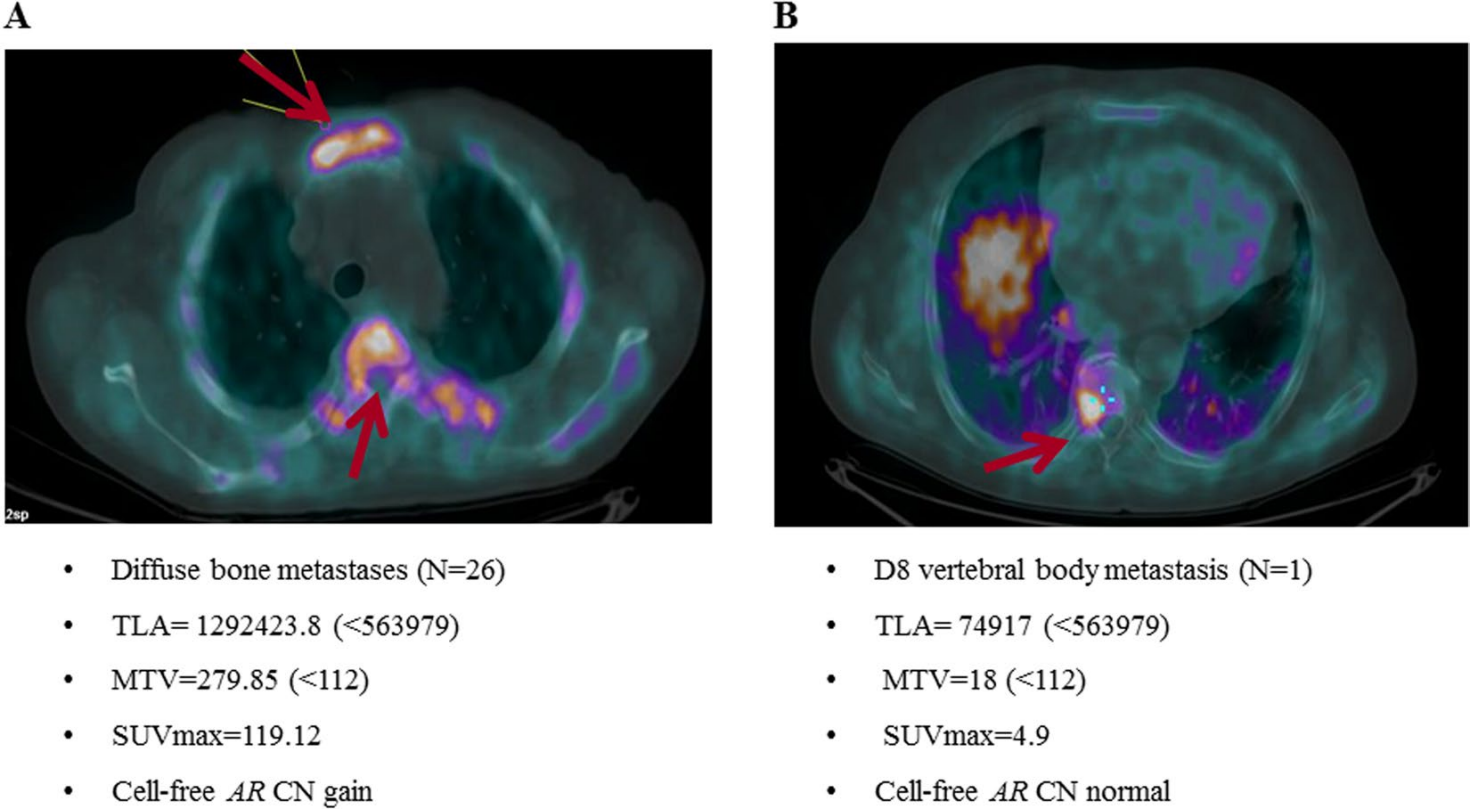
FCH-PET/CT of two metastatic CRPC patients. The FCH PET/CT image (A) demonstrates diffuse metabolically active skeletal metastases involving multiple vertebrae (arrows) with high values of TLA, MTV, SUVmax in one *AR*-gained patient. The FCH PET/CT image (B) shows involving one dorsal vertebrae (arrows) with high low values of TLA, MTV, SUVmax in one *AR*-normal patient.
